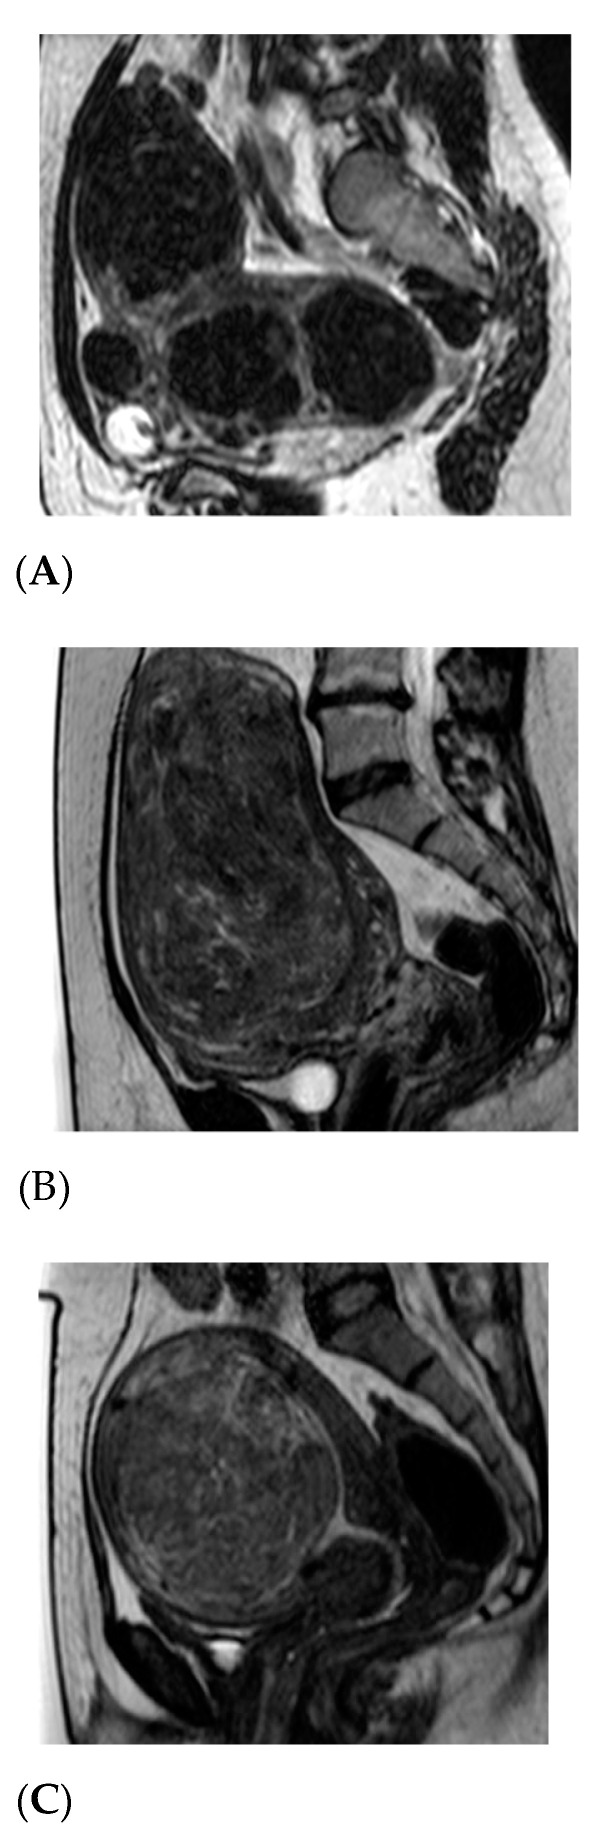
Magnetic resonance imaging (MRI) types of uterine fibroid (UF). (A). Type I presents as a “dark” UF as seen on MRI T2-weighted imaging. (B). Type II has a mixed MRI bright and dark structure. (C). Type III presents in MRI as a “bright” type of UF, usually not suitable for MRI-HIFU (high-intensity ultrasound) treatment.
